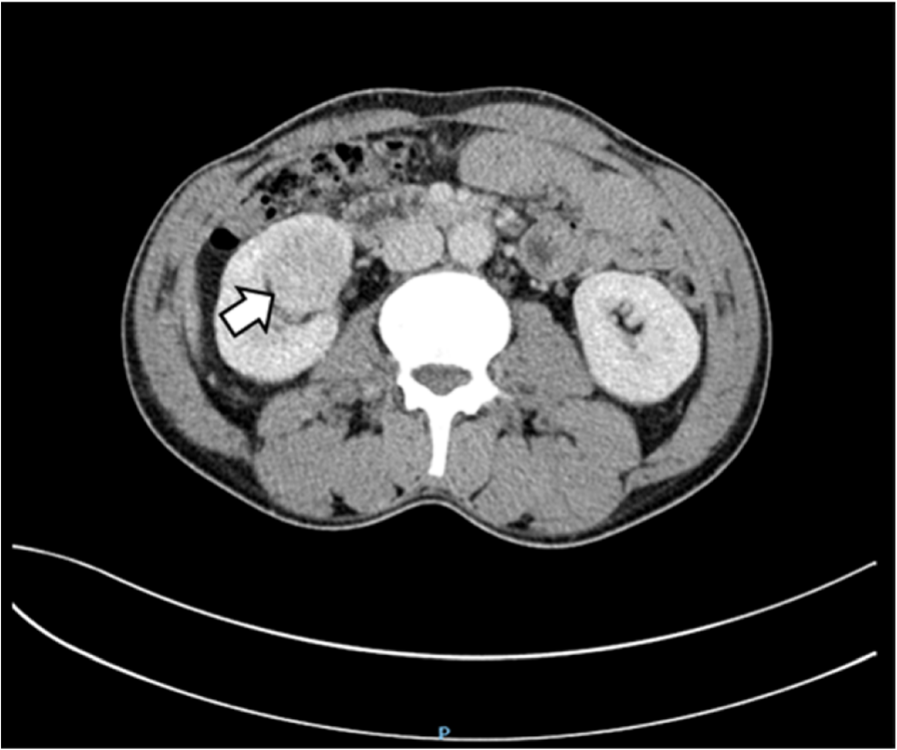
Contrast computed tomography scan shows mass in the right kidney. Arrow: kidney tumor
